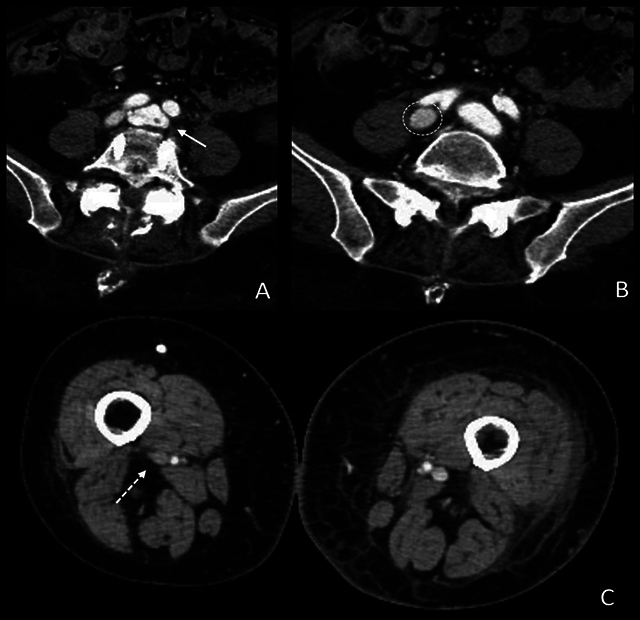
CT revealed arterial opacification of the left femoral vein and a swollen left lower limb.
